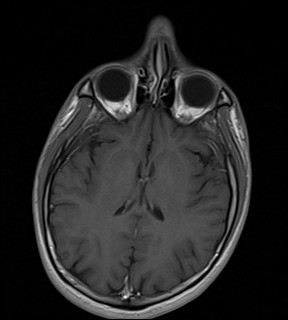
Gadolinium–enhanced axial brain MRI with T1 sequence.
